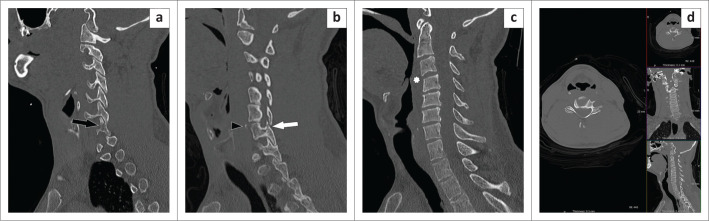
Paramidline sagittal (a and b) reconstructions of a cervical spine CT showing a fracture of the right inferior facet of the C6 vertebra (black arrow), a fracture of the right superior facet of the C7 vertebra (white arrow) and a fracture of the anterosuperior margin of the body of C7 (black arrowhead). A midline sagittal reconstruction (c) demonstrates reversed cervical spine lordosis but no listhesis. Associated prevertebral soft tissue swelling is present (white asterisk). Note that the patient was ‘scanned skew’ (d).
